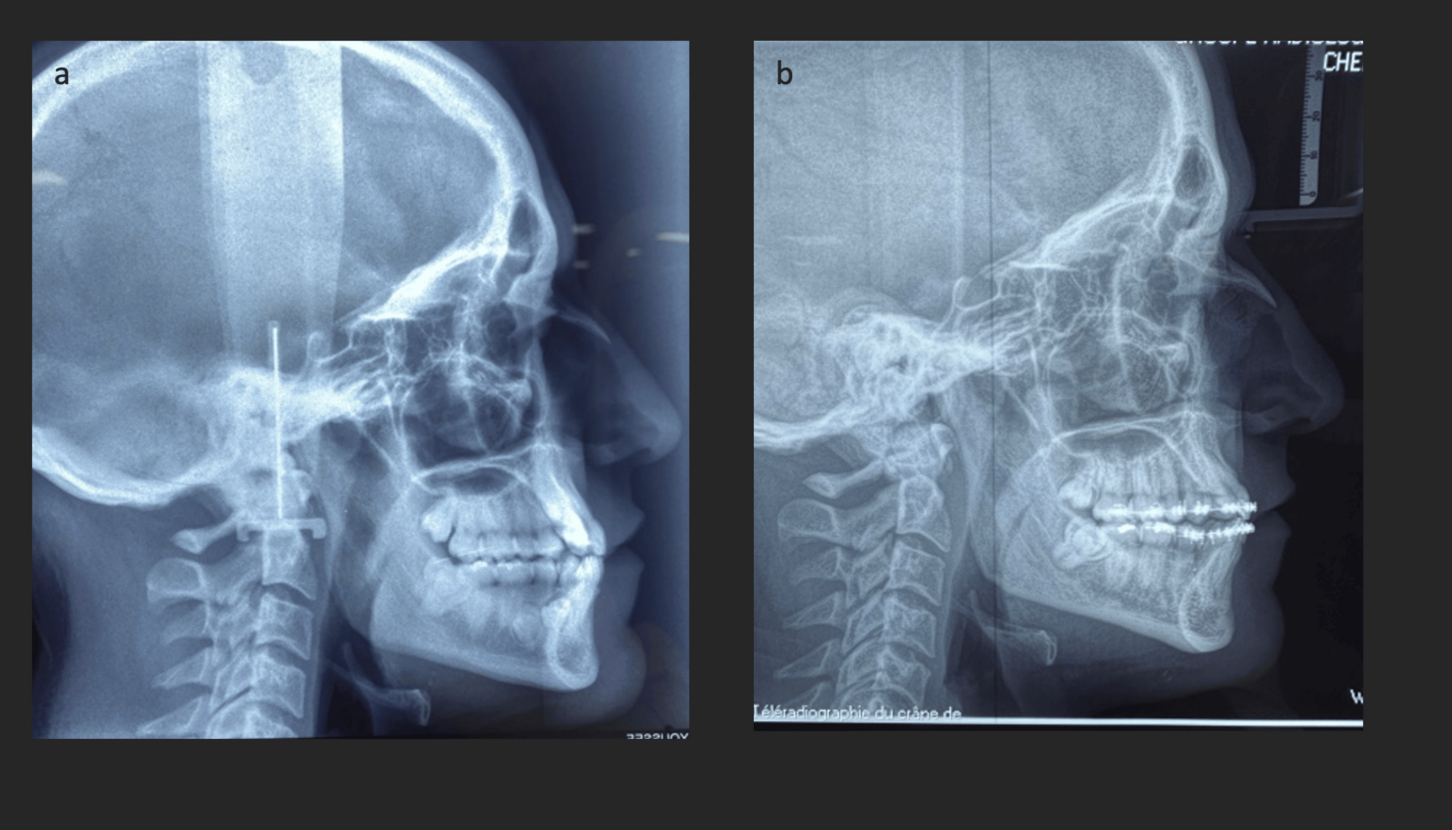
Lateral cephalometric radiographs; a: pretreatment and b: post-treatment.
